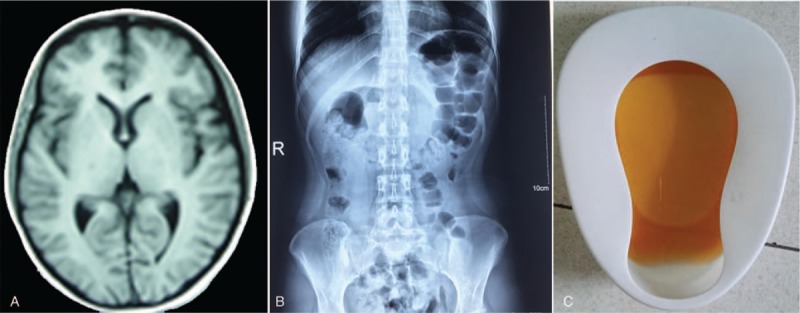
Clinical materials of the proband. (A) Brain magnetic resonance imaging (MRI): normal, (B) Abdomen X-ray: intestinal tympanites, (C) brown urine.
